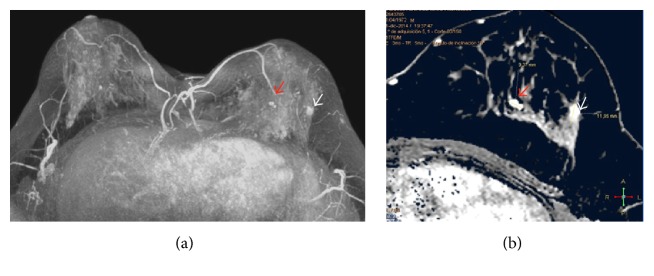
Breast Magnetic Resonance T1-weighted images, after gadolinium administration and axial maximum intensity projection (MIP) reformations. In addition to the previously biopsied lesion in the upper outer quadrant, there was another nodule 35 mm apart, in the upper inner quadrant. It measured 8 mm and was also submitted to US-guided biopsy: Rocha A, Servicio de Radiologia, Hospital Povisa, Vigo, Spain.
